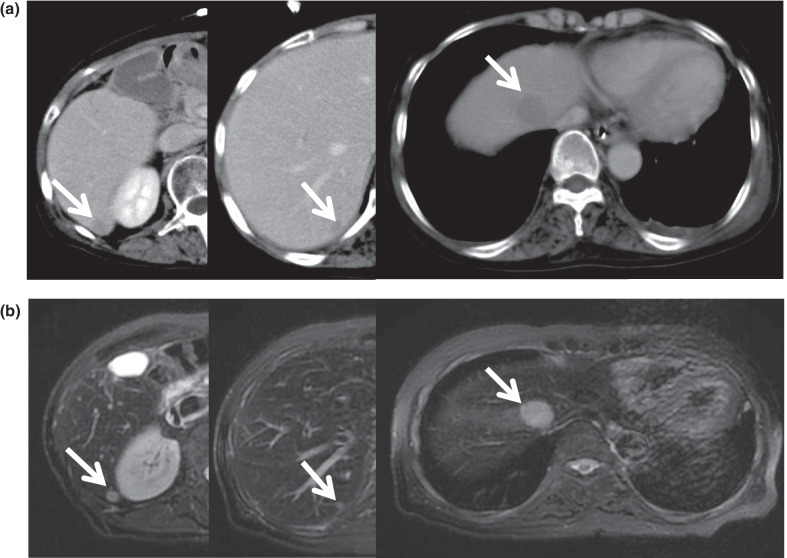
a, b Preoperative CT scan and MRI of the abdomen showing multiple liver metastases (white arrows). *CT* computed tomography, *MRI* magnetic resonance imaging
